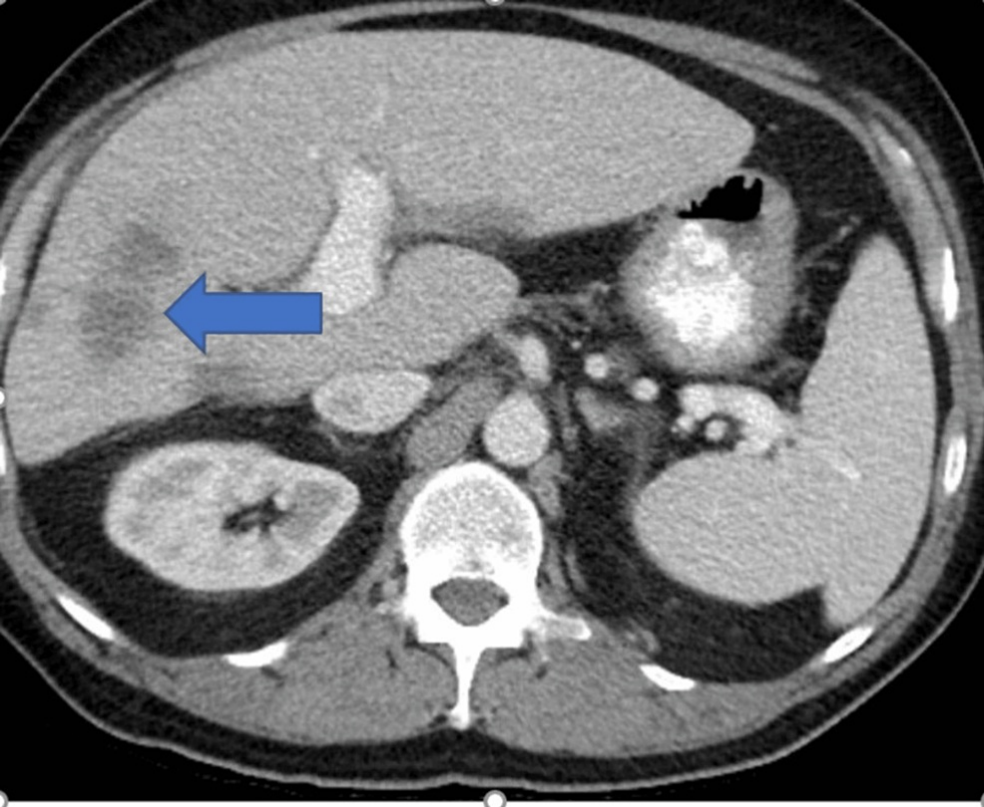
Contrast-enhanced CT of the abdomen (axial view) showing liver metastasis (blue arrow)
